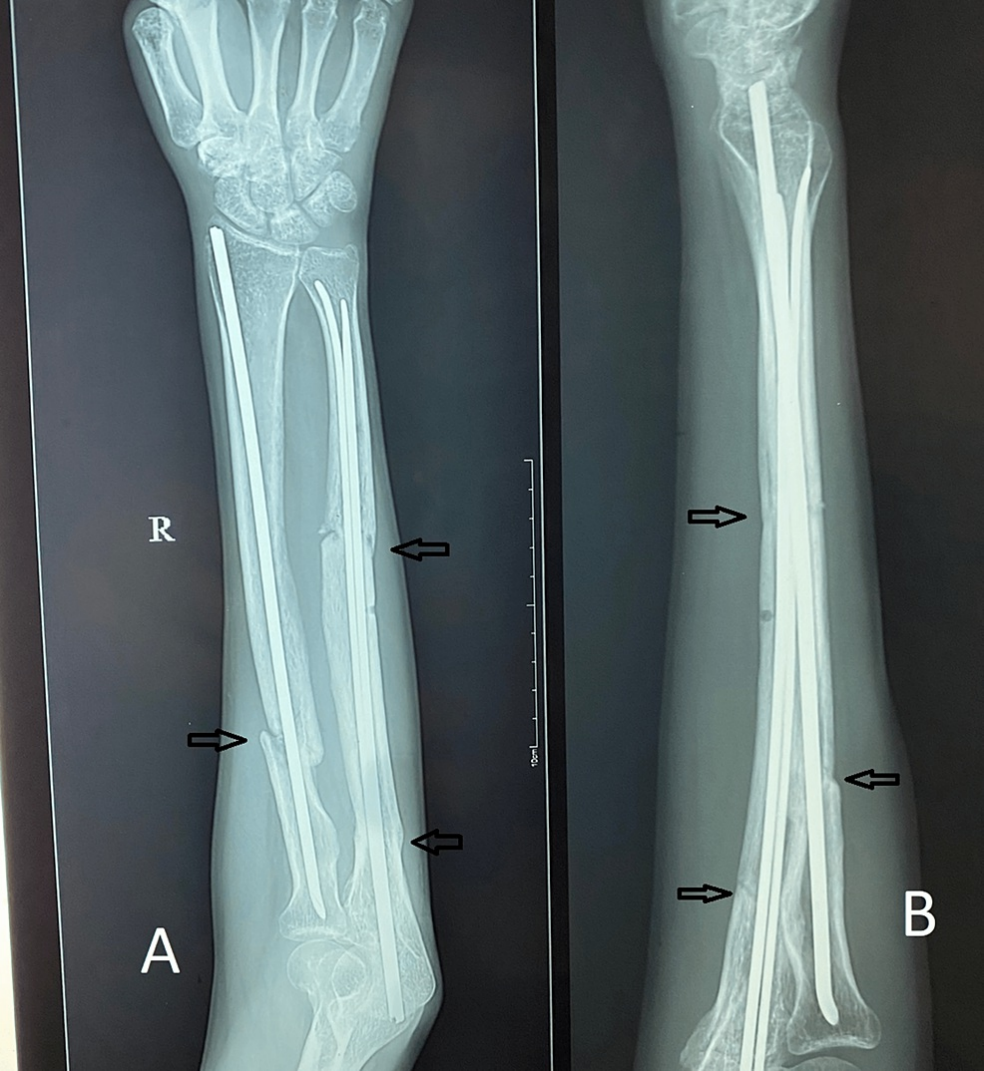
Three-month post-operative follow-up radiograph. Three-month follow-up radiograph showing progressive fracture union (marked with arrows). Figure A is the anteroposterior (AP) view, and figure B is the lateral view.
